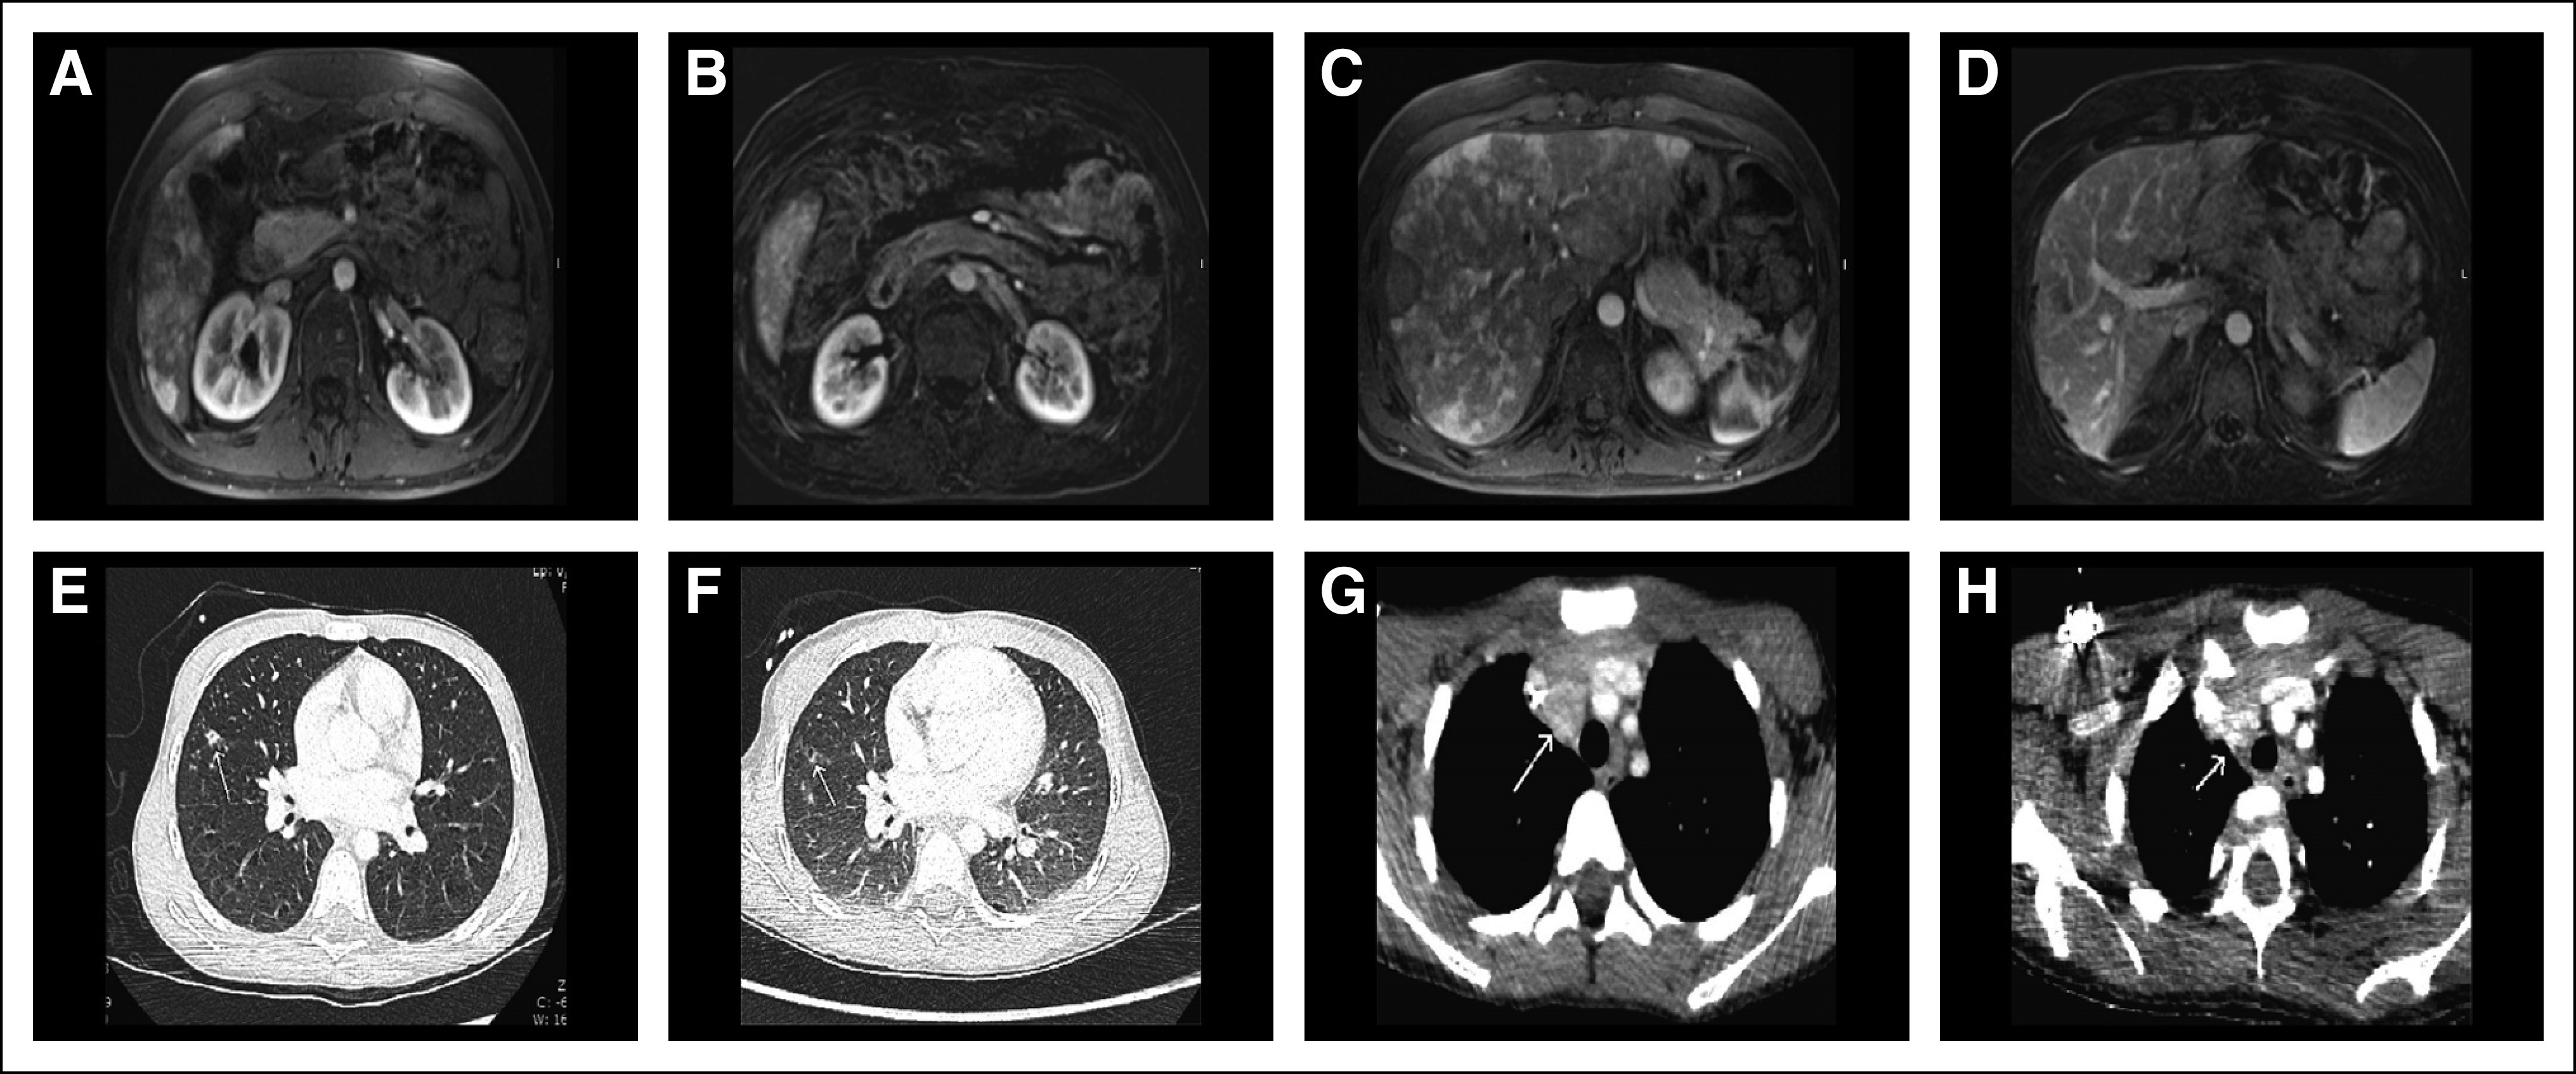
Selpercatinib activity in patients with medullary thyroid cancer. Patient 1: magnetic resonance imaging scans of (A) right lateral and (B) left anterior liver metastatic lesions at baseline and after 22 months of treatment with selpercatinib of (C) right lateral and (D) left anterior in a heavily pretreated patient with RET-mutated medullary thyroid cancer. A rapid improvement in symptoms and a partial response were reported after two cycles of treatment, which deepened over time. Patient 2: computed tomography scans at baseline and after 4 months of treatment with selpercatinib of thorax (E, F, respectively) and mediastinum (G, H, respectively) showing metastatic disease in a patient with *RET*-mutated medullary thyroid cancer. Early disease control was achieved after two cycles of treatment.
